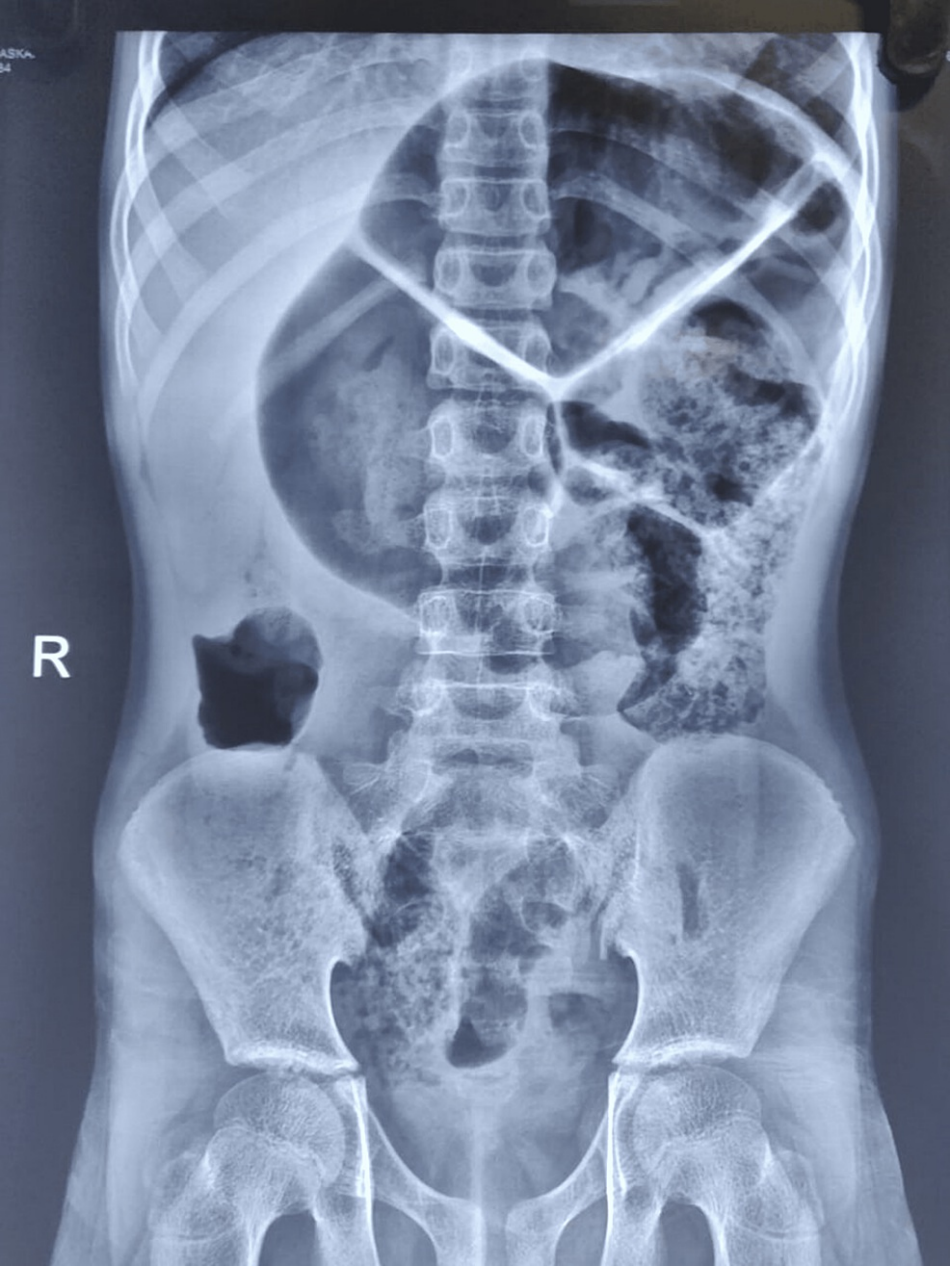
“Coffee bean sign” on X-ray abdomen erect
